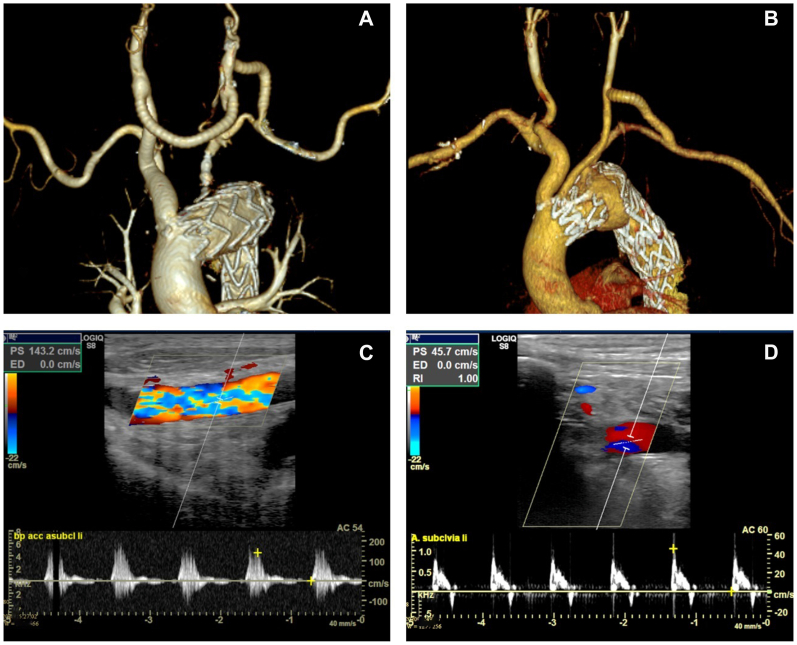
A, Three-dimensional (3D)-reconstruction of a computed tomography (CT)-angiography of a supra-aortic reconstruction with a ring-reinforced 8 mm polytetrafluoroethylene (PTFE) carotid-subclavian and a carotid-carotid bypass in a 78-year-old male patient with an aortic arch aneurysm who required endovascular repair. This CT-angiography has been performed 6 years and 3 months after the bypass reconstructions, currently showing no evidence of an anastomotic stenosis or bypass occlusion. B, 3D-reconstruction of a CT-angiography of a supra-aortic reconstruction with a ring-reinforced 8 mm PTFE carotid-subclavian bypass in a 78-year-old male patient with an aortic arch aneurysm. The CT-angiography has been performed 3 months after the bypass reconstructions, currently showing no evidence of an anastomotic stenosis or bypass occlusion. C, Triplex sonography of a supra-aortic reconstruction with a ring-reinforced 8 mm PTFE carotid-subclavian in a 52-year-old female patient with a thoracic aortic aneurysm and an aneurysm of the aortic arch, showing the carotid-subclavian bypass with a triphasic Doppler waveform and a flow of 1.43 m/s in the bypass. D, Triplex sonography of a supra-aortic reconstruction with a ring-reinforced 8 mm PTFE carotid-subclavian in a 52-year-old female patient with a thoracic aortic aneurysm and an aneurysm of the aortic arch, showing the restored perfusion of the subclavian artery after the arterial reconstruction with a triphasic Doppler waveform and a flow of 0.46 m/s in the distal subclavian artery.
